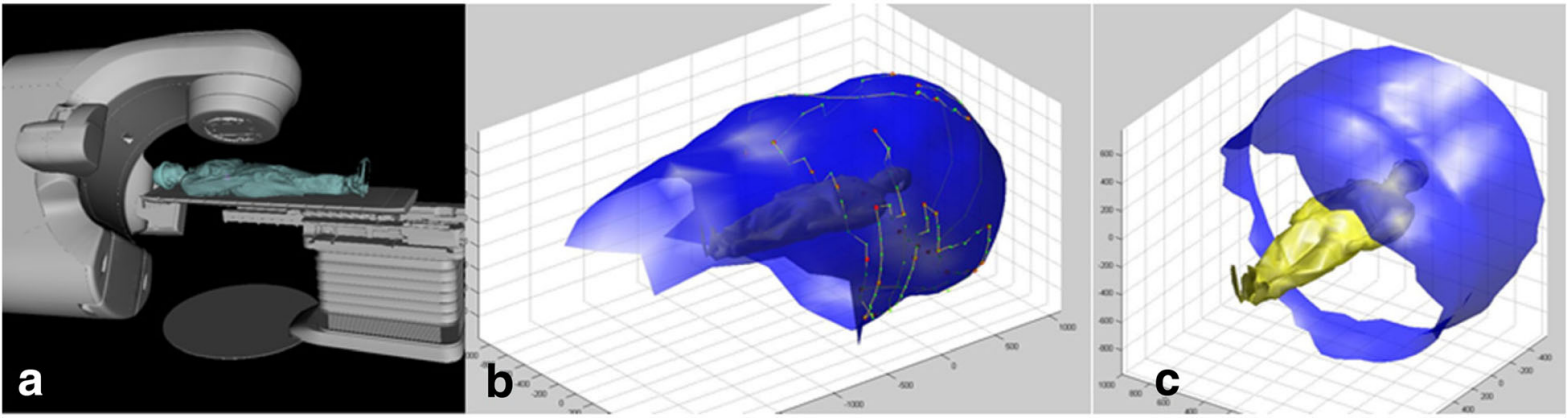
Accessible non-coplanar beam geometry for a left lung target based on the actual machine model and a 3D laser scanned human surface shown in (a). b The non-coplanar surface that can be accessed by the source when up to 120 cm source-to-isocenter distances are used by enabling additional couch shifts. c The non-coplanar surface that can be accessed by the X-ray source for treatment limited to 100 cm isocentric set up. The surface only accounts for a point source and that the effective coverage will be larger due to the typical 40 cm field-of-view
